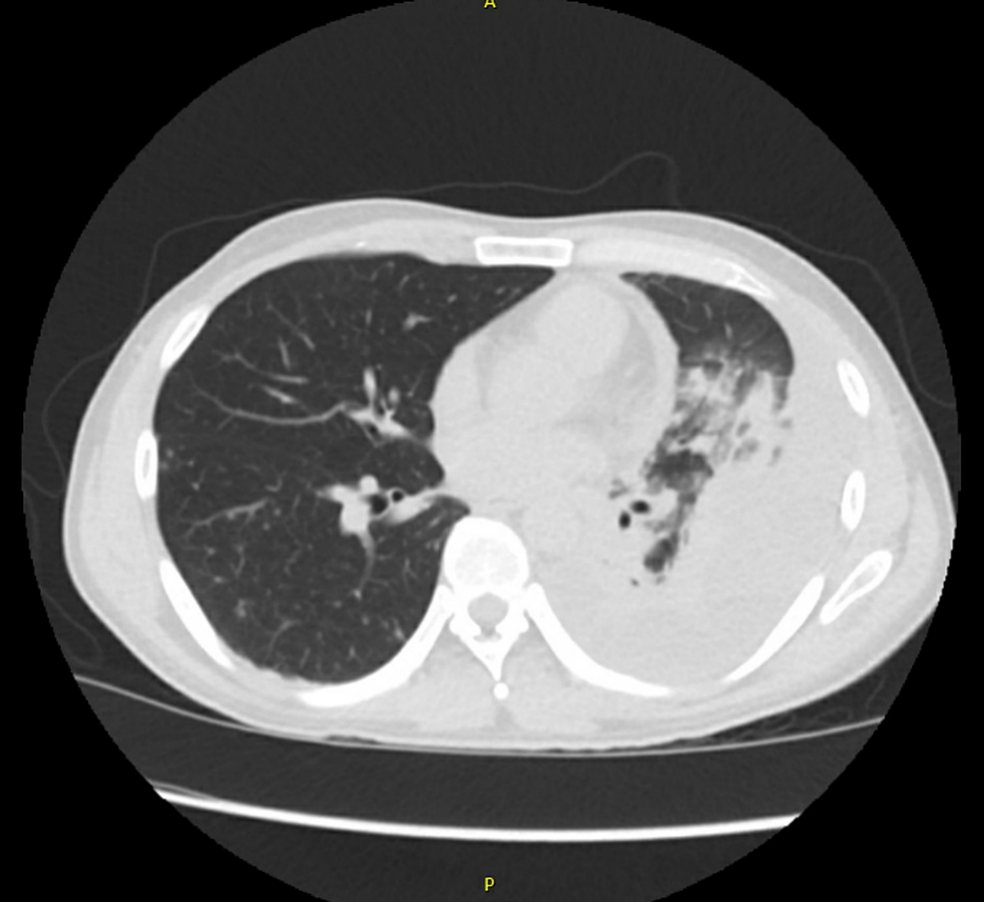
CT chest showing left pleural effusion with associated consolidation.
